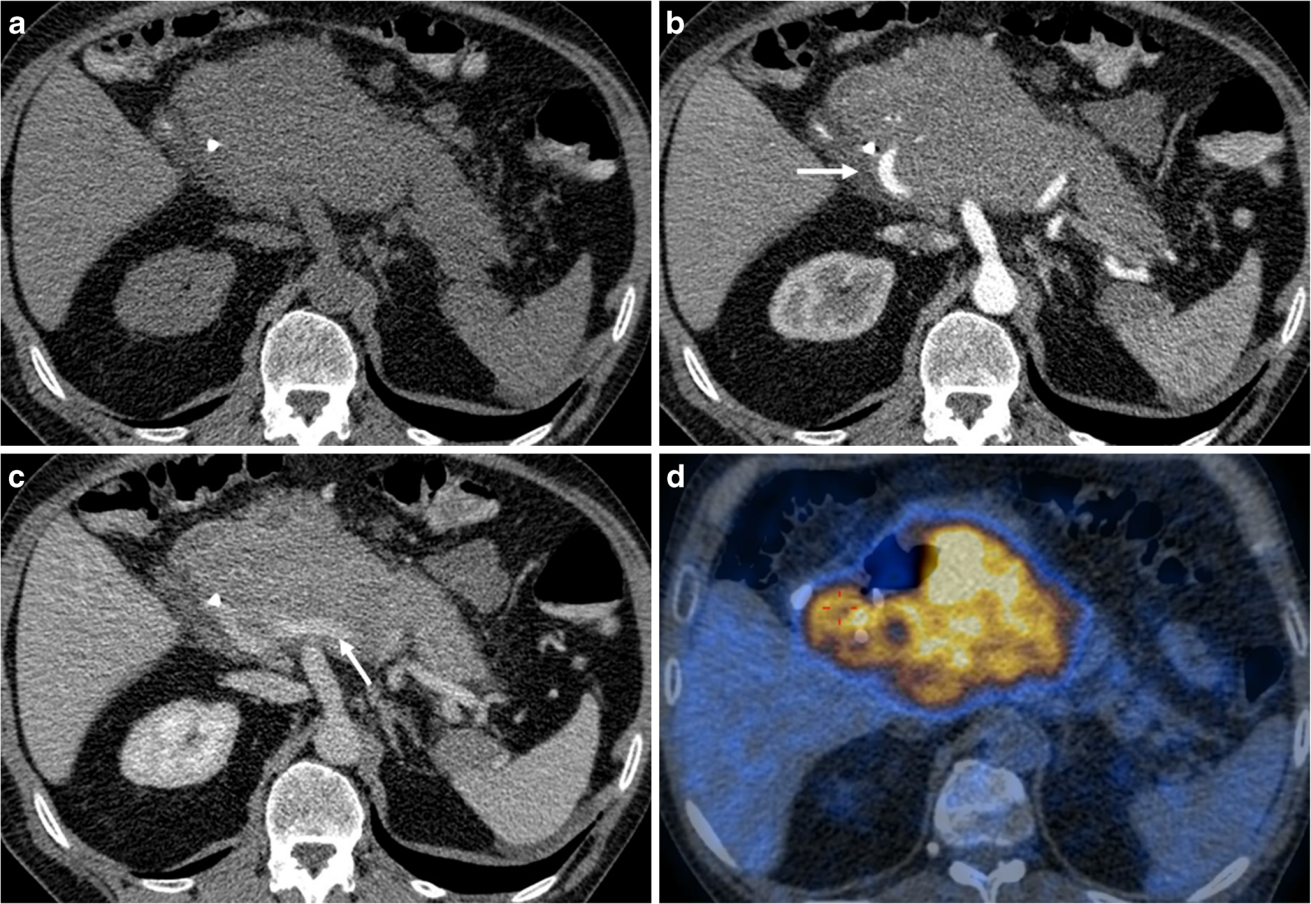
A 51-year-old male patient with primary pancreatic lymphoma, histotype diffuse large B-cell (Ki 67 score > 90%). a CT scan of the abdomen shows the presence of a large solid mass in the body-head of the pancreas. A biliary stent is seen. b, c CT images after contrast medium administration in the arterial-pancreatic phase (a) and portal-venous phase (b): the arteries and veins (arrows) are encased but not infiltrated. The main pancreatic duct is not enlarged. d This ^18^FDG PET-CT fusion image reveals high metabolic activity in the lesion
